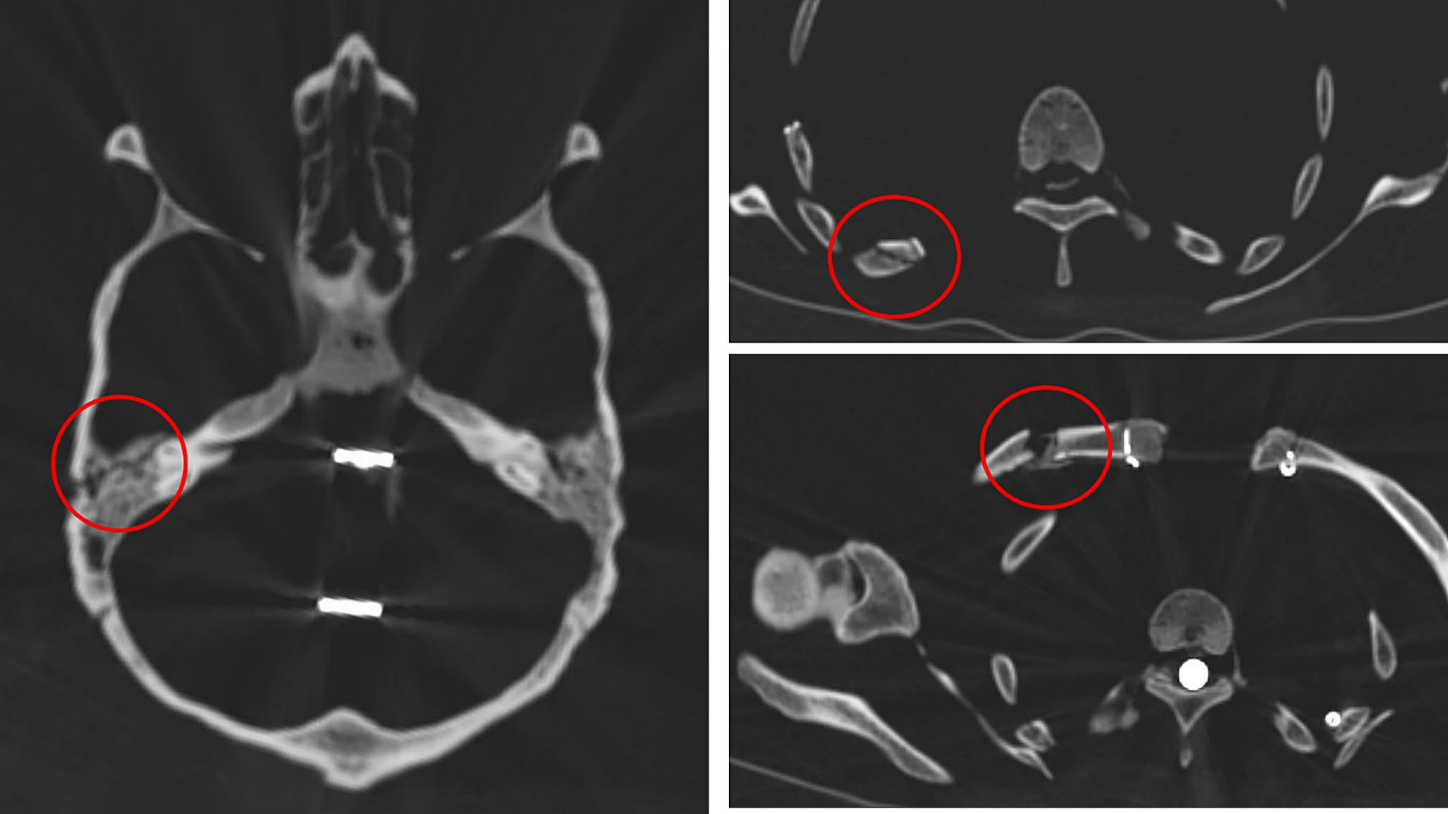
CT-scans of the skull trauma with lesion of the os petrosum (left), rib fracture (upper right) and the clavicle fracture (lower right)
